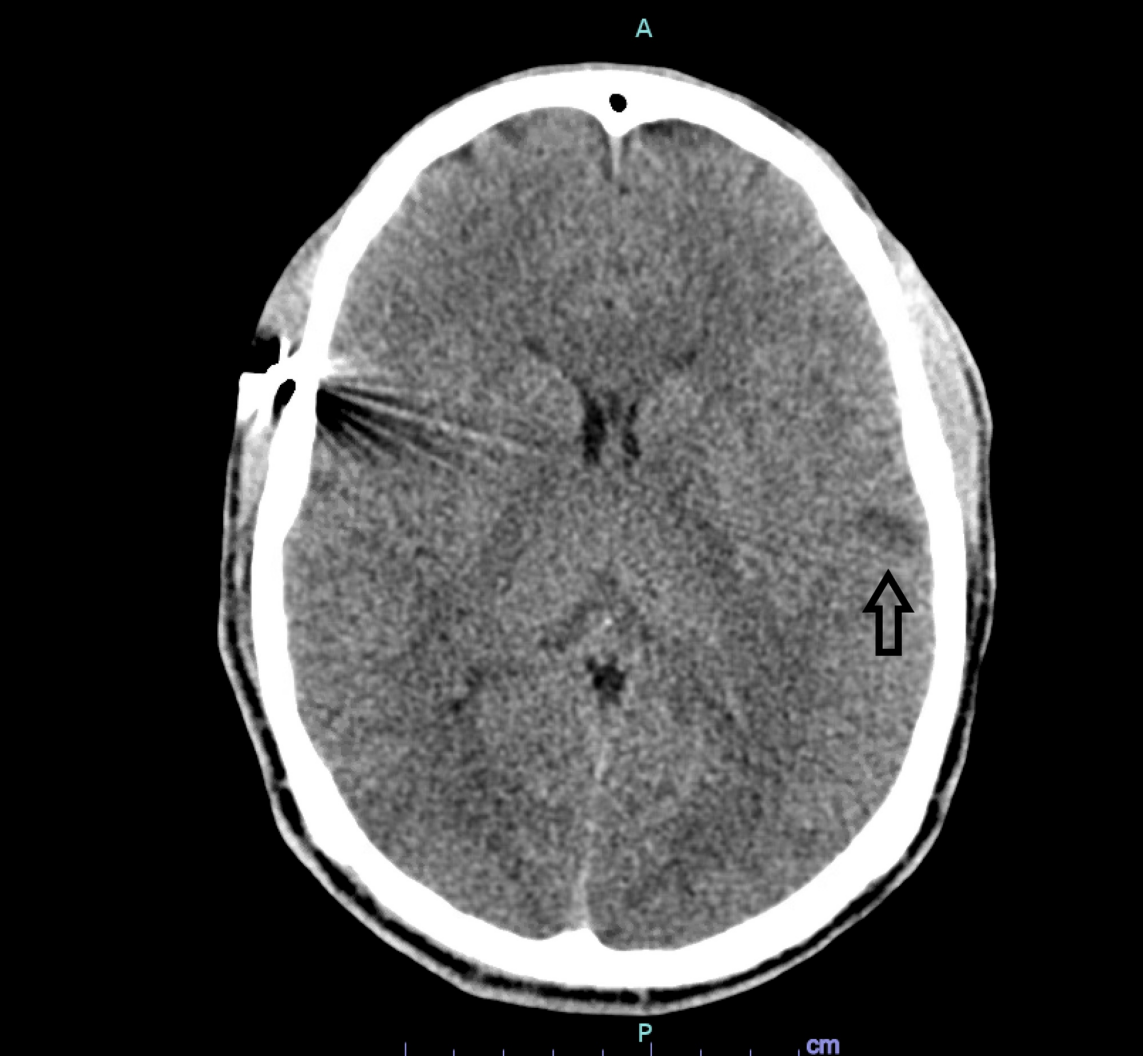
CT head with new area of hypodensity in the left frontal lobe
